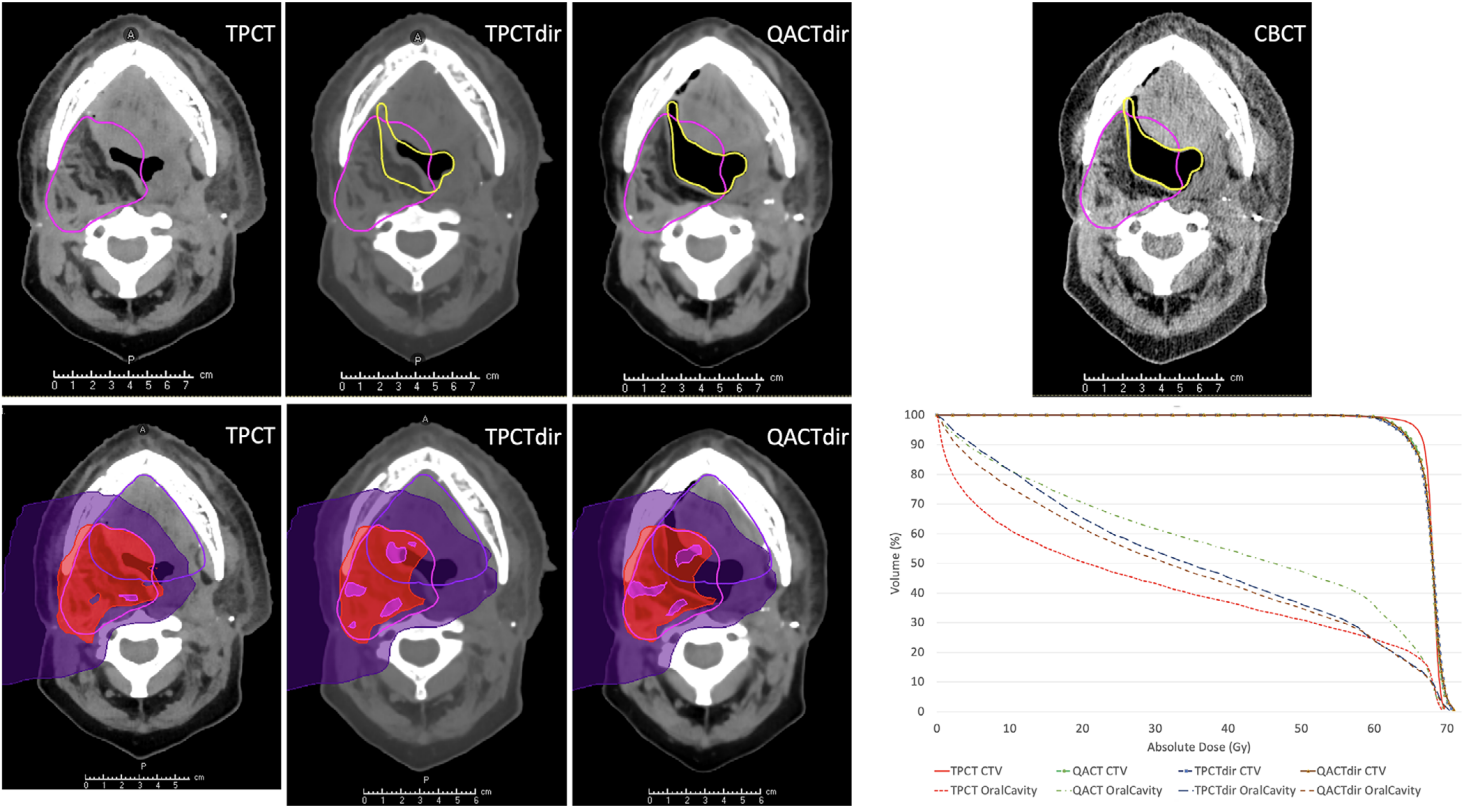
Shrinkage of graft and its effect on the oral cavity change. Treatment planning CT (TPCT), deformed TPCT to CBCT (TPCTdir), QACTdir, and the cone beam CT (CBCT) on top. The corresponding dose in the bottom (except for CBCT). The DVH for the clinical target volume (CTV) and the oral cavity are shown as well. Both QACTdir and the CBCT represent correct anatomy changes, while the TPCTdir failed to reflect the graft shrinkage. With density override to the air cavity contour derived from the CBCT, the dosimetric evaluation based on the TPCTdir matches that based on the QACTdir well. For demonstration purpose only, the CTV and oral cavity contours are rigidly copied (instead of deformed) from the TPCT to TPCTdir and QACTdir image sets, while the air cavity (yellow contour) is copied from CBCT to TPCTdir and QACTdir image sets
